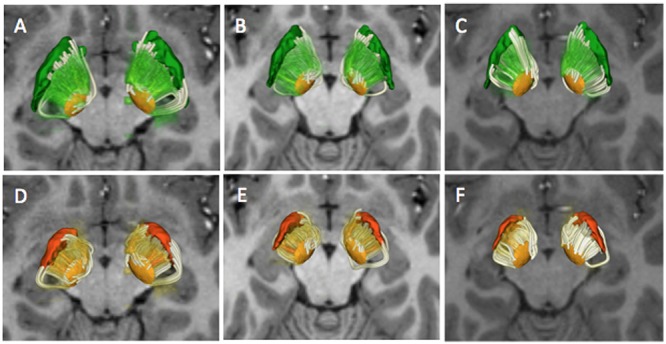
Combined deterministic and probabilistic tractography reconstructions. The figure shows a superior 3D view of the deterministic and probabilistic tracts interconnecting the STN and the GPe (top row) and STN and GPi (bottom row) in three subjects (A–D: HCP 100408; B–E: HCP:101915; C–F: HCP 103414). The MC-det tracts are represented as streamlines (white) and the CSD-prob tracts are represented as volume-rendered imaged (green, orange). The tracts are superimposed on an axial T1-weighted image at the level of the cerebral peduncles with 3D surface models of the STN (light orange), GPi (dark orange), and GPe (green).
